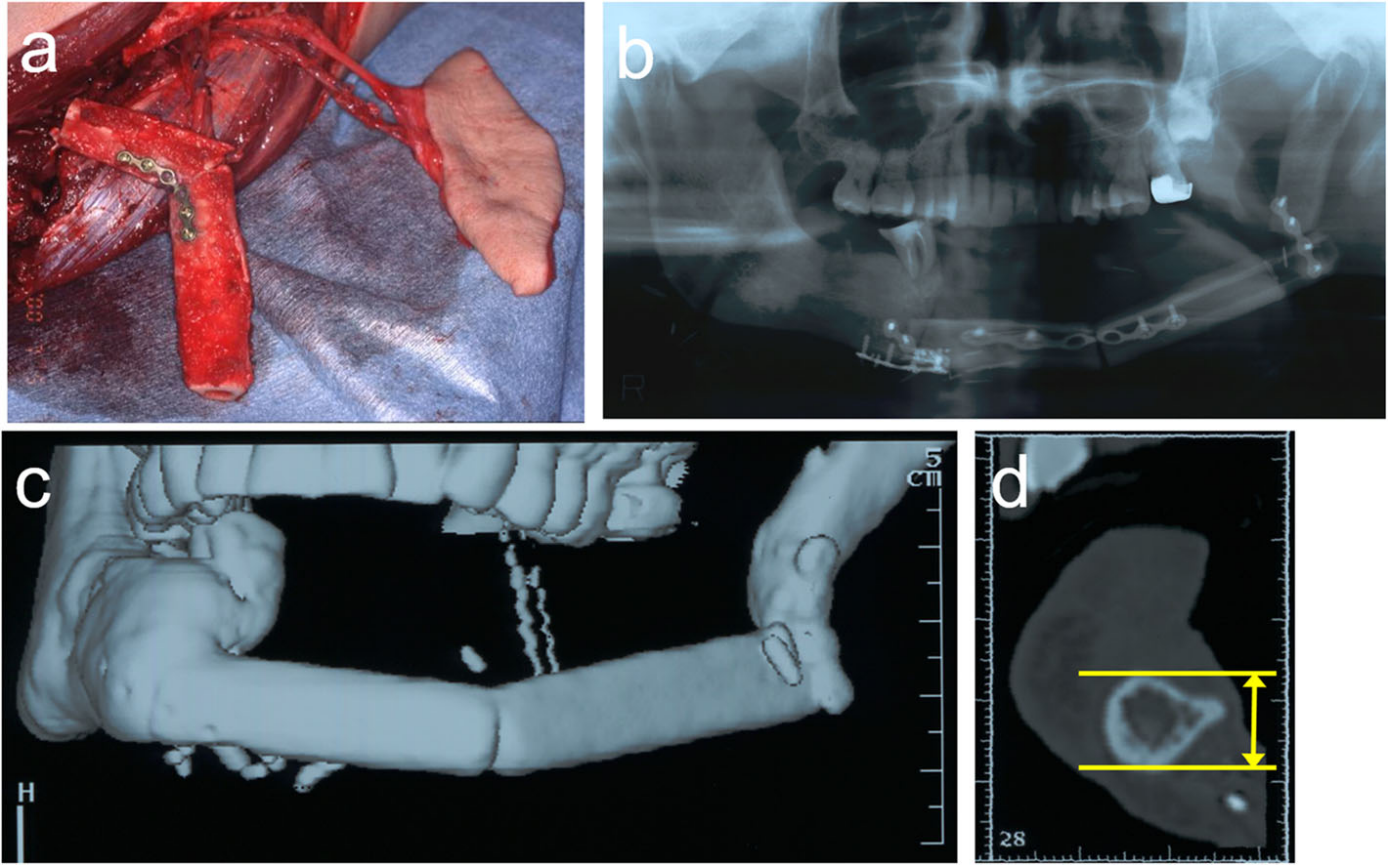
a A free vascularized fibula flap. b Orthopantomogram after mandibular reconstruction with a free vascularized fibula flap. c 3-D computed tomography scan and d cross sectional image 1 year after the mandibular reconstruction with a free vascularized fibula flap. The height of the fibula was 12 mm
